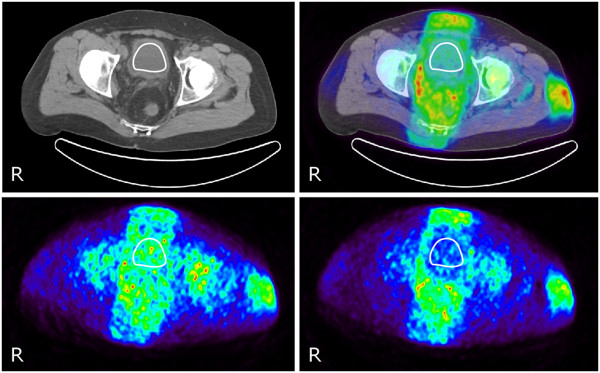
Transaxial sections of the ACCT (left) and PET/CT (right) (0 to 30 min). The image shows the VOI drawn in the urinary bladder (upper row). Lower row shows the transaxial section of the PET image acquired from 0 to 2 min (left) and from 25 to 30 min (right) along with the VOI imposed in the urinary bladder. During this time period, the bladder activity representing ^15^O has almost completely declined while the surrounding soft tissue activity, mainly representing ^11^C, is still clearly visible.
